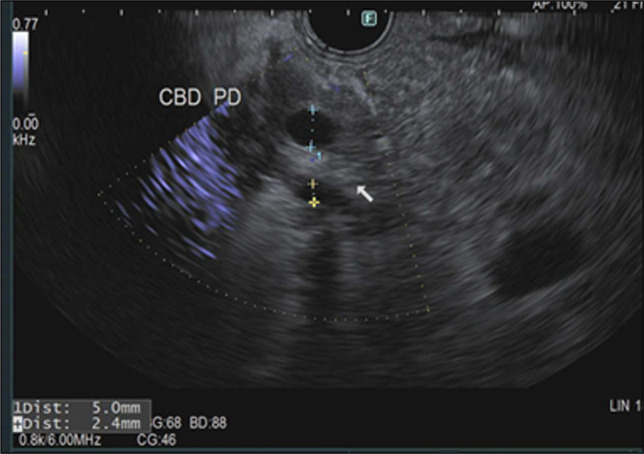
Endoscopic ultrasound image demonstrating a normal common bile duct measuring 5 mm. CBD, common bile duct; PD, pancreatic duct.
